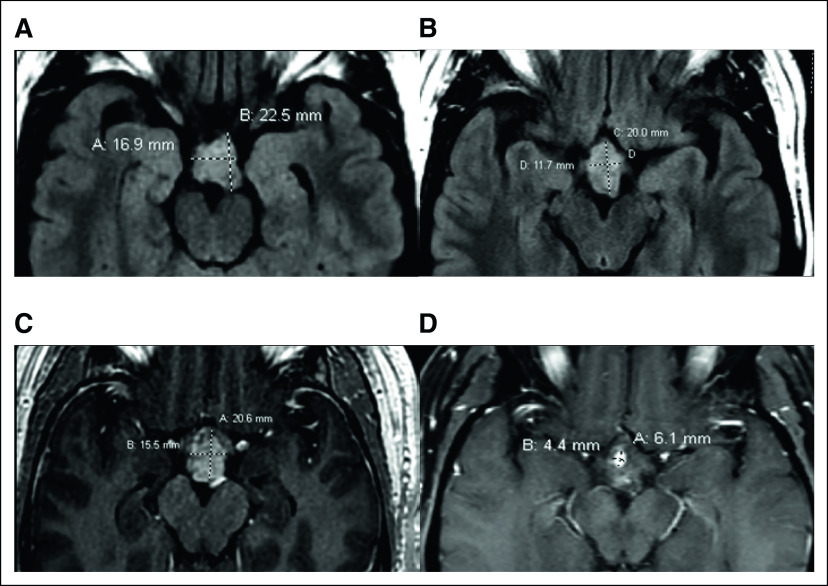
Baseline MRI images after intensity-modulated radiation therapy and near gross total resection of hypothalamic pilocytic astrocytoma. (A) Axial FLAIR and (B) postcontrast images demonstrating the residual enhancing tumor. After 10 months of treatment, hypothalamic tumor still demonstrates (C) good response with a significant reduction in tumor size in axial FLAIR and (D) only minimal residual focus of enhancement present in postcontrast MR images. MRI, magnetic resonance imaging.
